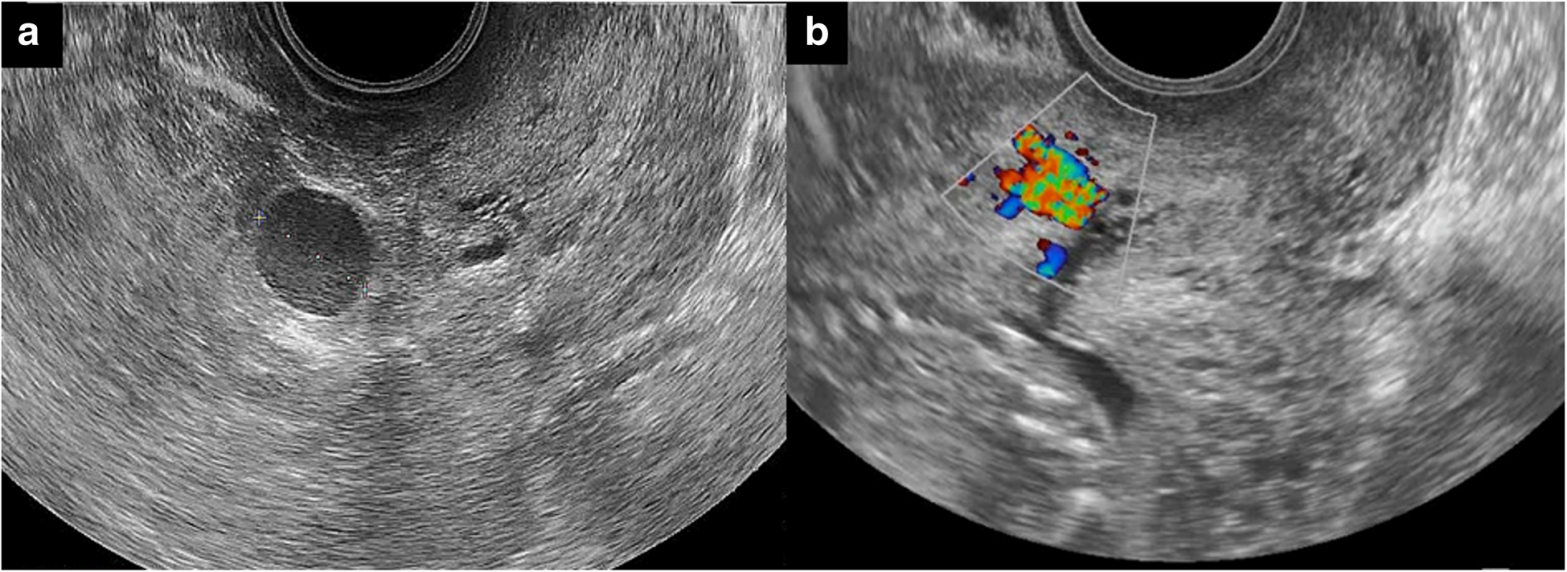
a Hypo-echoic coin lesion measuring 17.5 mm in diameter surrounded by hetero-echoic mass. Transvaginal ultrasonography. b Spinning turbulent flow pattern inside the coin lesion. Transvaginal color Doppler ultrasonography
